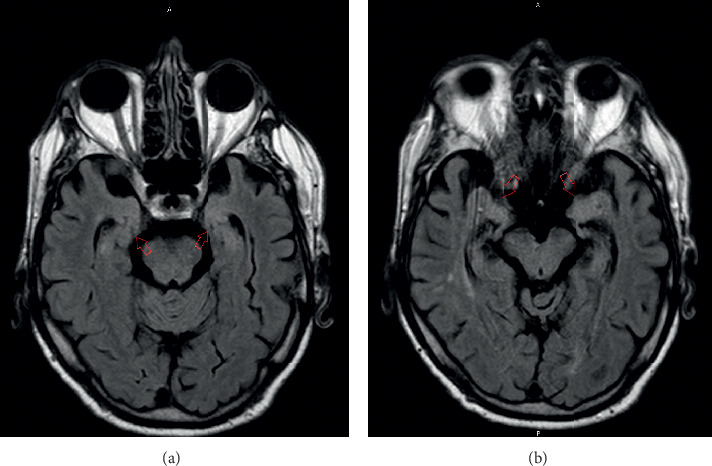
((a) and (b)) Axial FLAIR MRI showing slight increased signal in bilateral temporal lobes.
